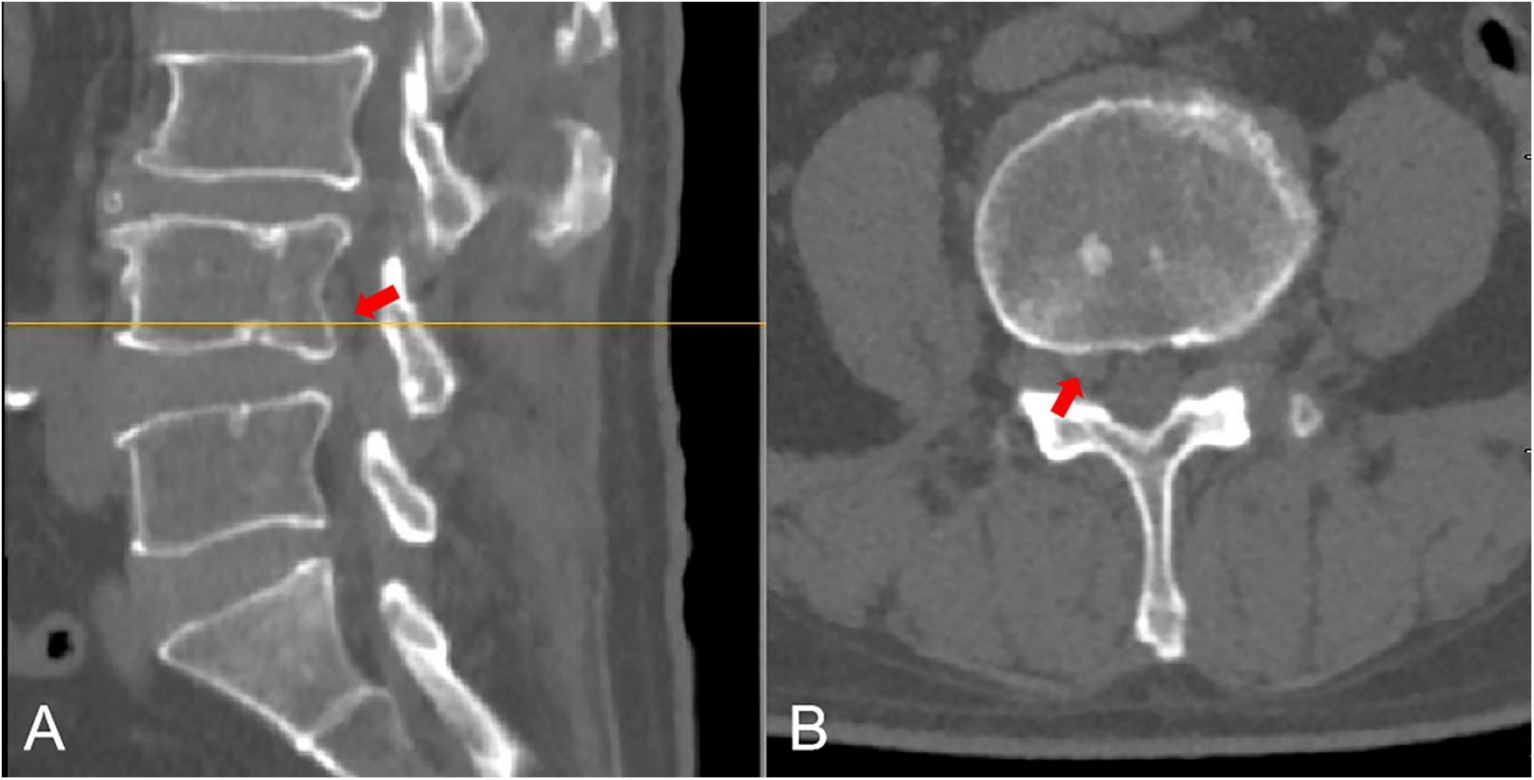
Preoperative CT revealed an L4/5 disc extrusion (indicated by a red arrow); (A) L4/5 disc extrusion had migrated superiorly. (B) Axial image demonstrated a significant right-sided disc herniation at the L4/5 level.
